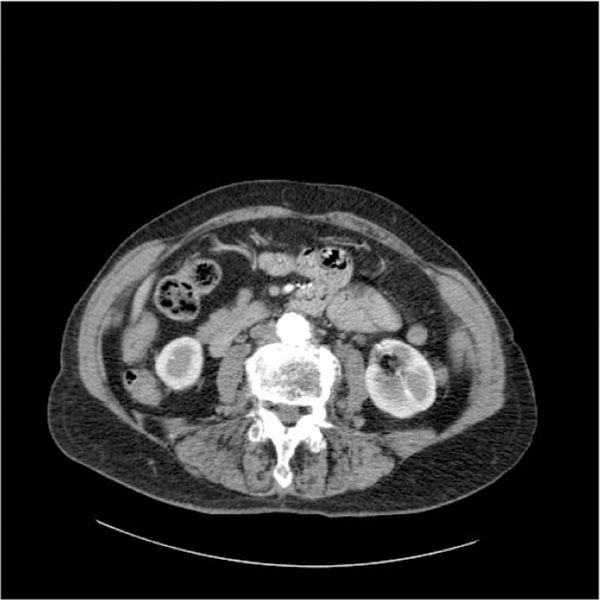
A computed tomography scan angiogram 1 year after endovascular aortic repair showing no evidence of aortitis or leak.
